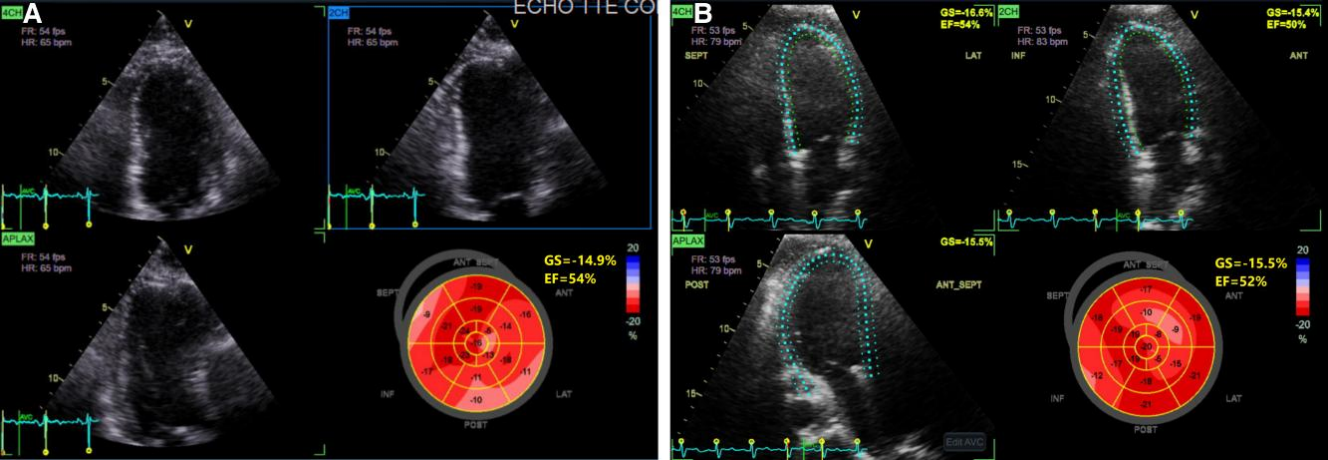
(*A*) The baseline echocardiogram had an estimated ejection fraction reported of 55%–60% with a global longitudinal strain measurement of 14.9%. (*B*) Admission echocardiogram showed an estimated ejection fraction of 55% (in-line video 1) with no regional wall motion abnormalities and a global longitudinal strain of −15.8%.
